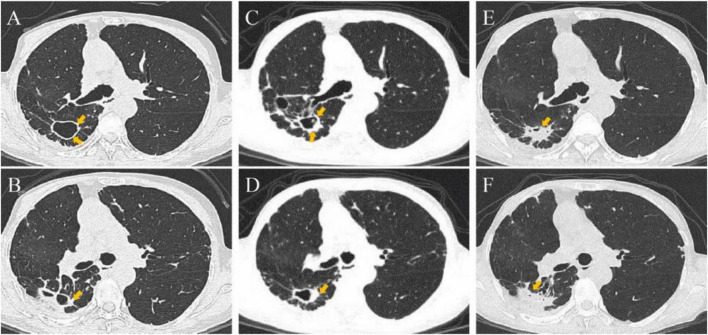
This patient’s follow-up chest CT images for suspected *Aspergillus* infection. (A,B) Multiple cavities remain in the right lung due to tuberculosis. (C,D) The cavity walls of the posterior segment of the right upper lobe and the dorsal segment of the right lower lobe thickened in December 2022. (E,F) Contents appeared in the cavities of the right lung in March 2023.
